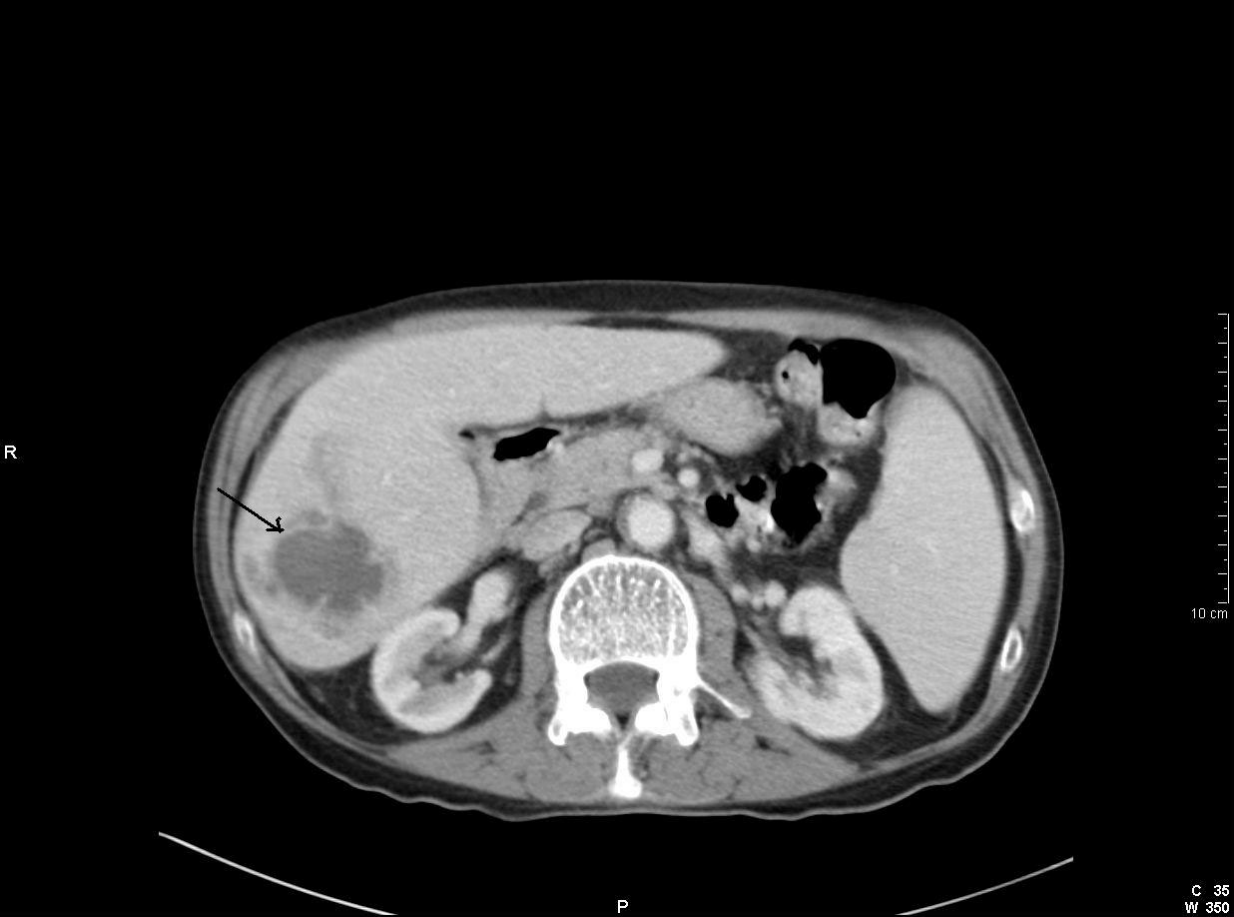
CT scan demonstrating several ill-defined low attenuation lesions with some peripheral enhancement.
